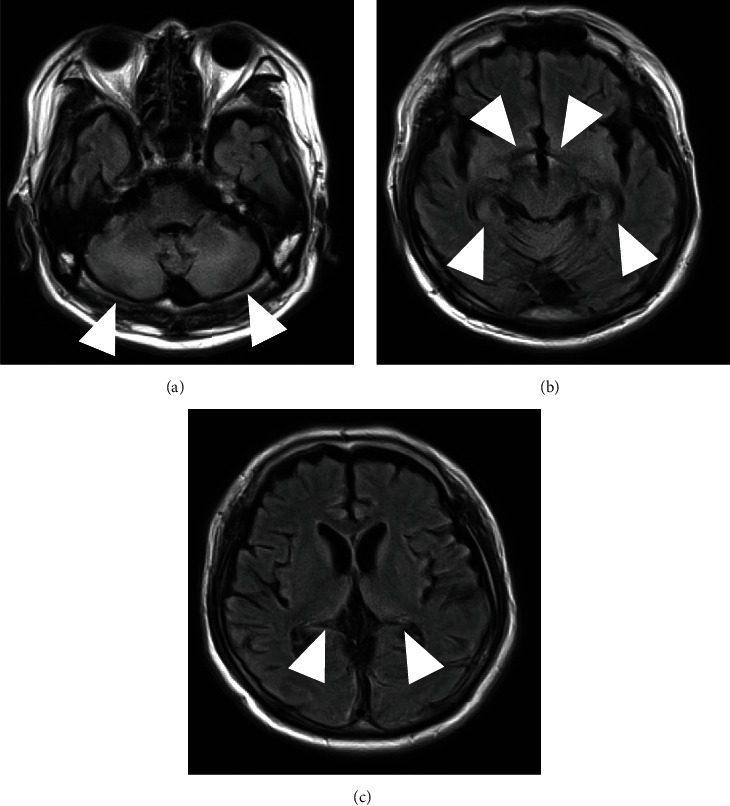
MRI on day 57 revealed symmetrical hyperintensity in the bilateral pons, cerebellar hemispheres, hippocampus, thalamus, and hypothalamus on FLAIR. (a) Cerebellar hemispheres (arrow). (b) Hippocampus and hypothalamus (arrow). (c) Thalamus (arrow). FLAIR: fluid-attenuated inversion recovery; MRI: magnetic resonance imaging.
